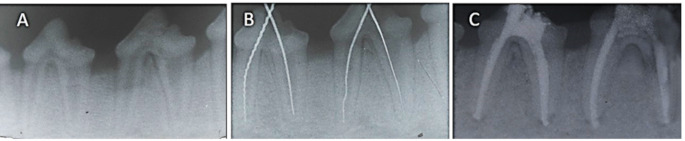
(A) Preoperative periapical radiographs were obtained to verify the apical closure of roots, evaluate the health of the periapical tissue, and establish the provisional working length. (B) Using radiography to determine and verify the working length. (C) Postoperative radiograph
